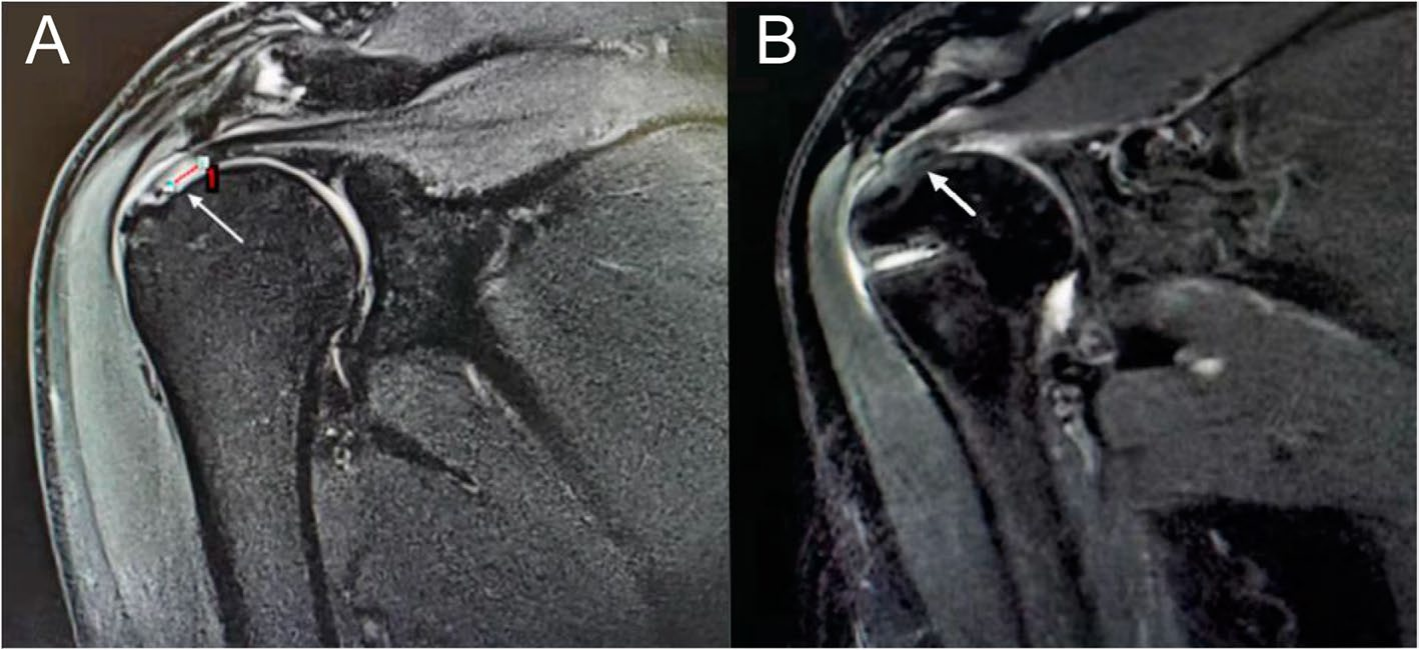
Preoperative and postoperative MRI scans of a patient who had partial articular supraspinatus tendon avulsion. A Preoperative MRI of a PASTA lesion. B MRI at the last follow-up after repair of a PASTA lesion using the threading lasso fixation technique MRI, magnetic resonance imaging.
